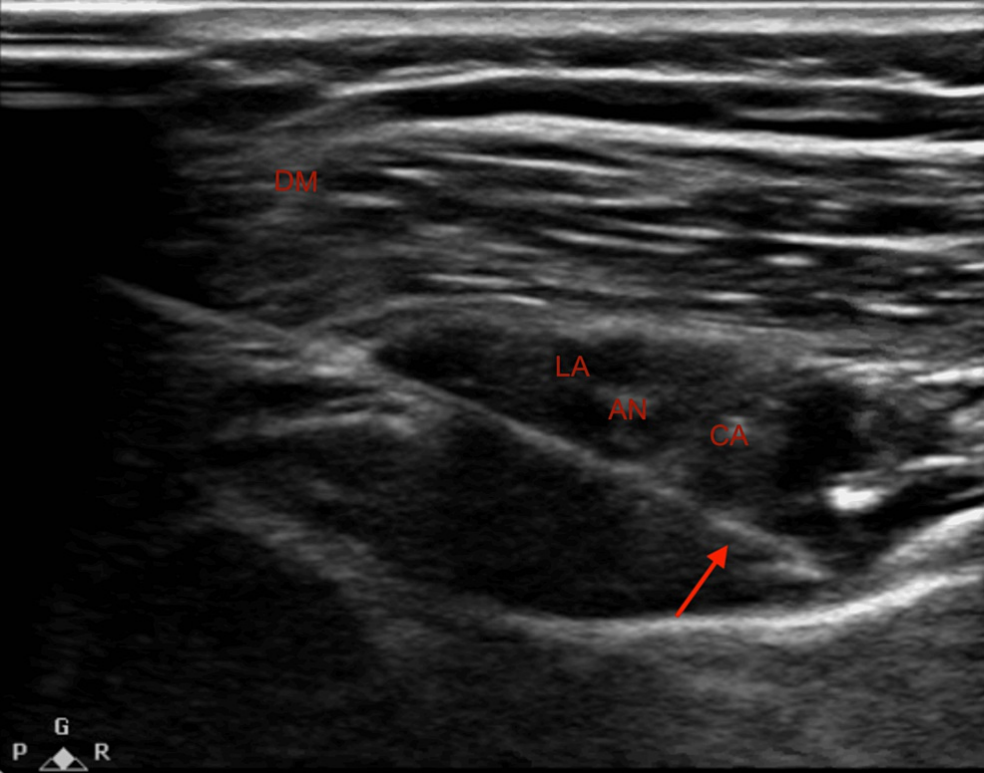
Ultrasound-guided needle placement for the axillary nerve block. DM: deltoid muscle, AN: axillary nerve, CA: circumflex artery, LA: local anesthetic, Arrow: needle tip
